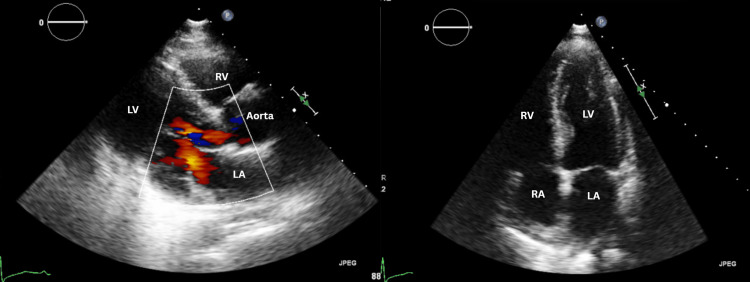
Color doppler images Parasternal long-axis view with color doppler (image on the left) showing normal flow across the left ventricular outflow tract extending into the ascending aorta. Apical four-chamber view (image on the right) demonstrating normal chamber orientation with preserved left ventricular cavity size. The right atrium (RA), right ventricle (RV), left atrium (LA), and left ventricle (LV) are labeled. No pericardial effusion can be seen. Regional wall motion abnormalities involving the mid-inferior, apical anterior, apical septal segments, and the apex were identified on dynamic echocardiographic assessment and are not fully appreciable on representative static images.
